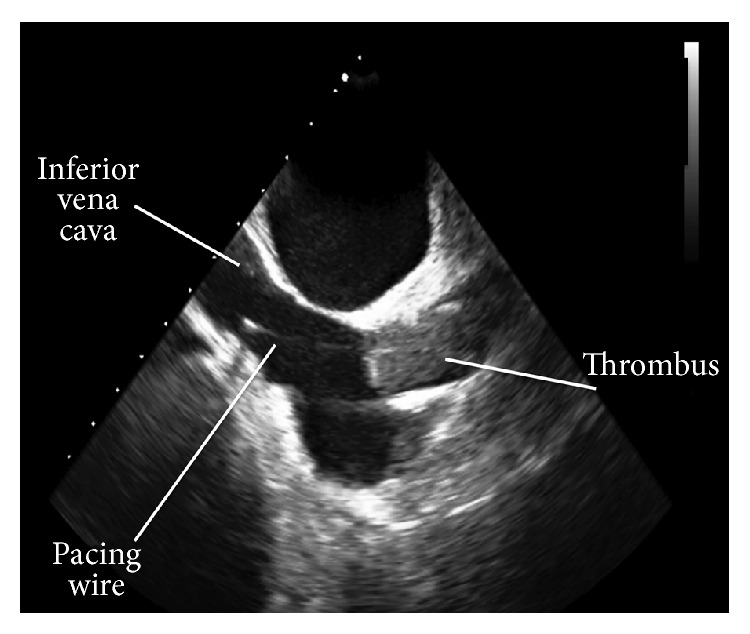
Transesophageal echocardiogram demonstrating a large thrombus in the superior vena cava. The pacing wire appears to overlay the thrombus. Because this is a 2D image, hence no spatial depth, it is unclear if there is a space between the thrombus and wire or whether it is independent of this.
